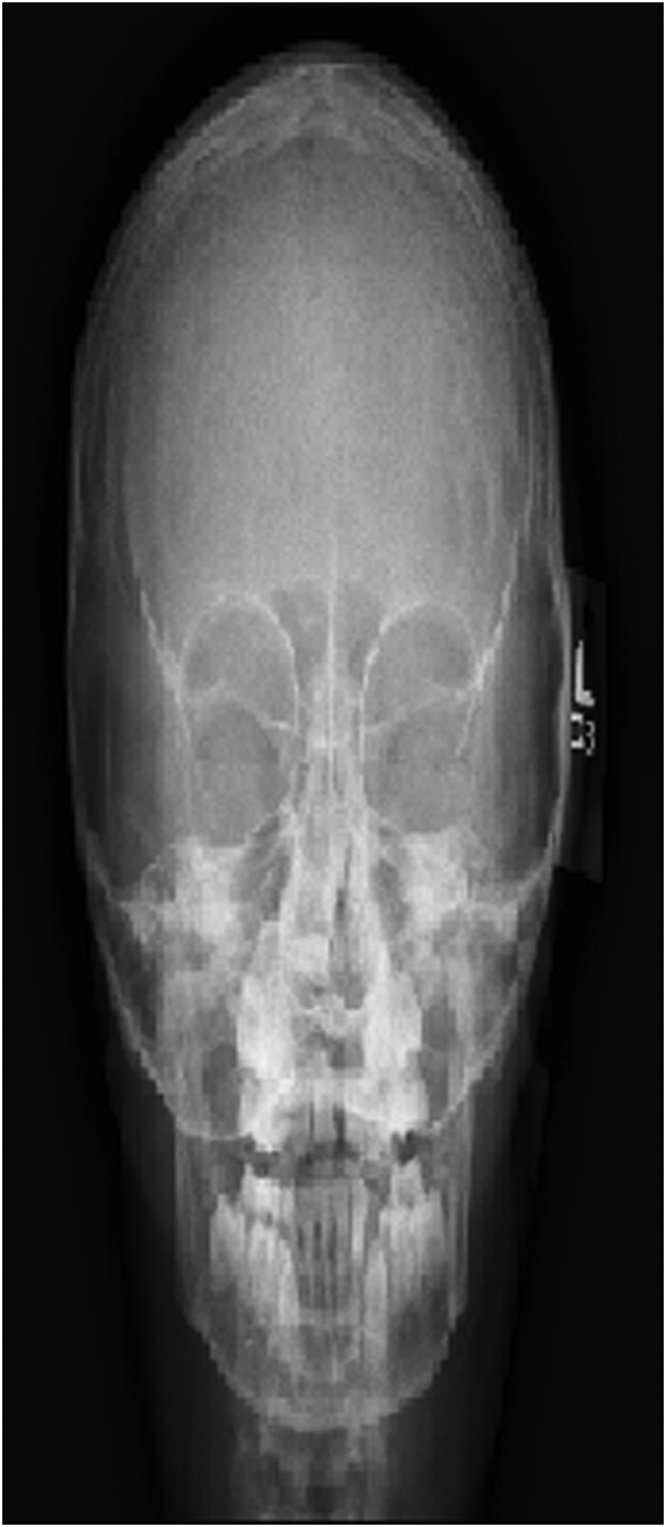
Plain x-ray (AP view) of the skull revealing radiopaque mass filling the right nasal cavity.
